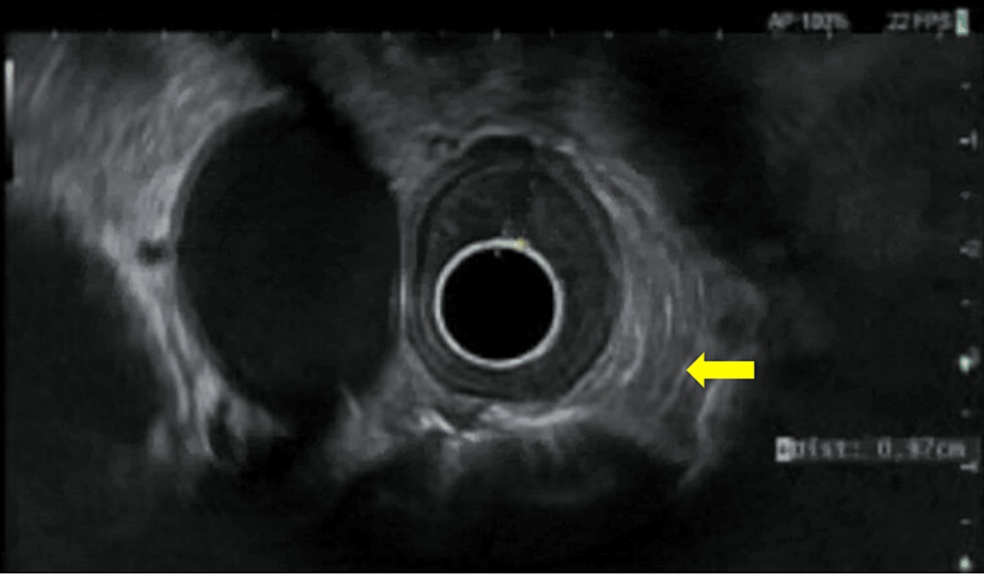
Endoscopic ultrasound revealing gastroesophageal junction mass (arrow) with invasion into muscularis propria, consistent with known adenocarcinoma from previous pathology, stage T3N1Mx
